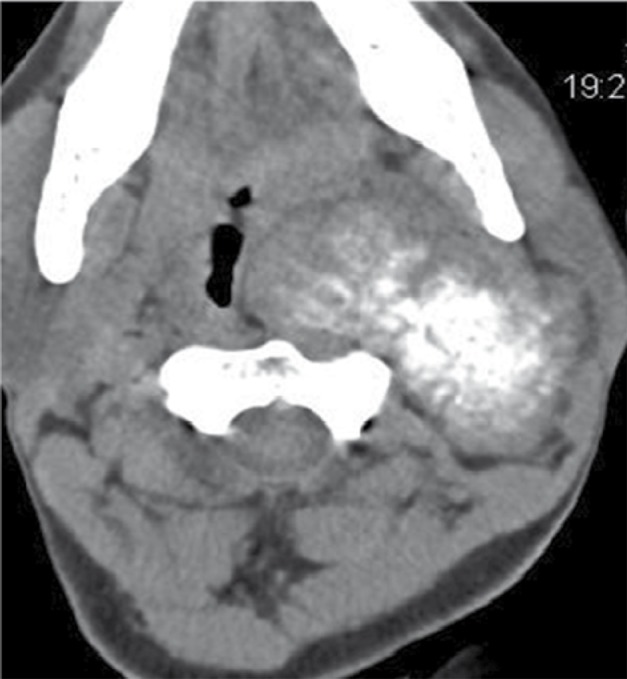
Plain CT Scan, Axial Section Revealed a Well-Defined Soft Tissue Density Mass Lesion in the Left Parapharyngeal Region With Central Dense Amorphous Calcifications.
